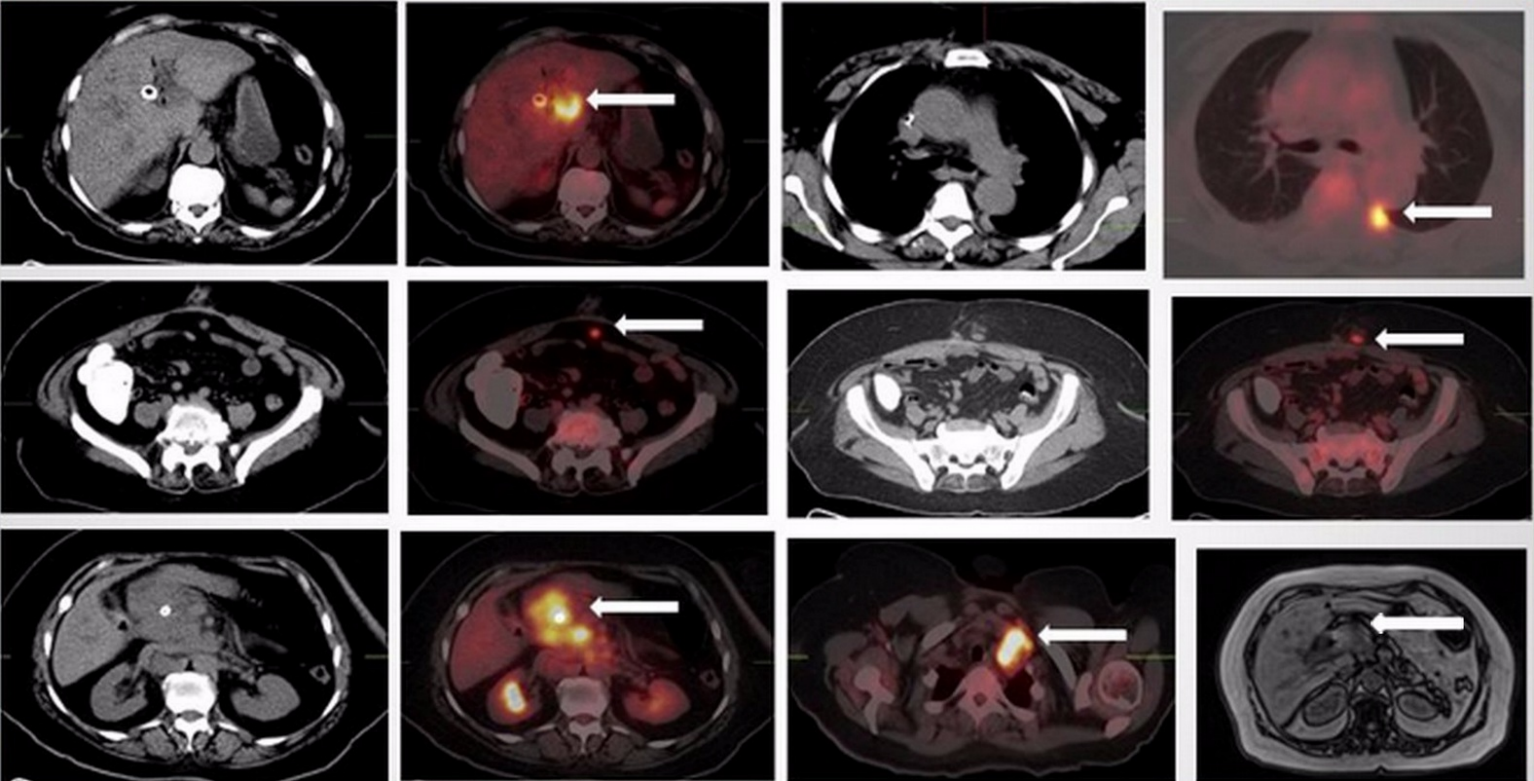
FDG-PET/CT and abdominal MRI PET/CT images demonstrate an irregular hypodense left hepatic lobe lesion, right posterior pleural-based density, deposits in the midline anterior abdominal cavity adjacent to the umbilical port site, soft tissue density in the subcutaneous tissue of the anterior abdominal wall superficial to the umbilicus, a poorly-circumscribed infiltrative epigastric mass with a centrally located biliary stent and multiple lymph nodes in the left supraclavicular station with increased FDG uptake (see arrows). The abdominal coronal T1-weighted VIBE MR images demonstrate a low-intensity left hepatic lobe lesion that is also seen as an infiltrative mass on axial MR images (see arrow).​
